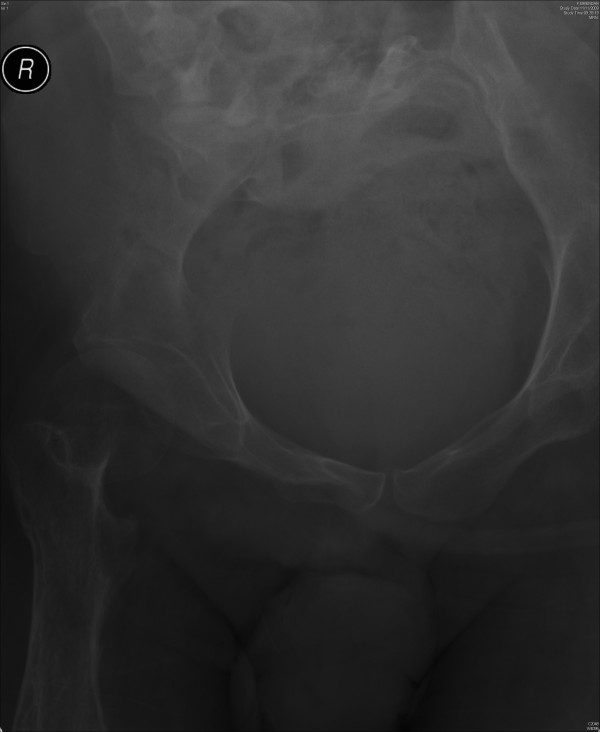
X-ray of pelvis, taken on 11 November 2009, showed soft tissue shadow of distended urinary bladder in pelvis.
